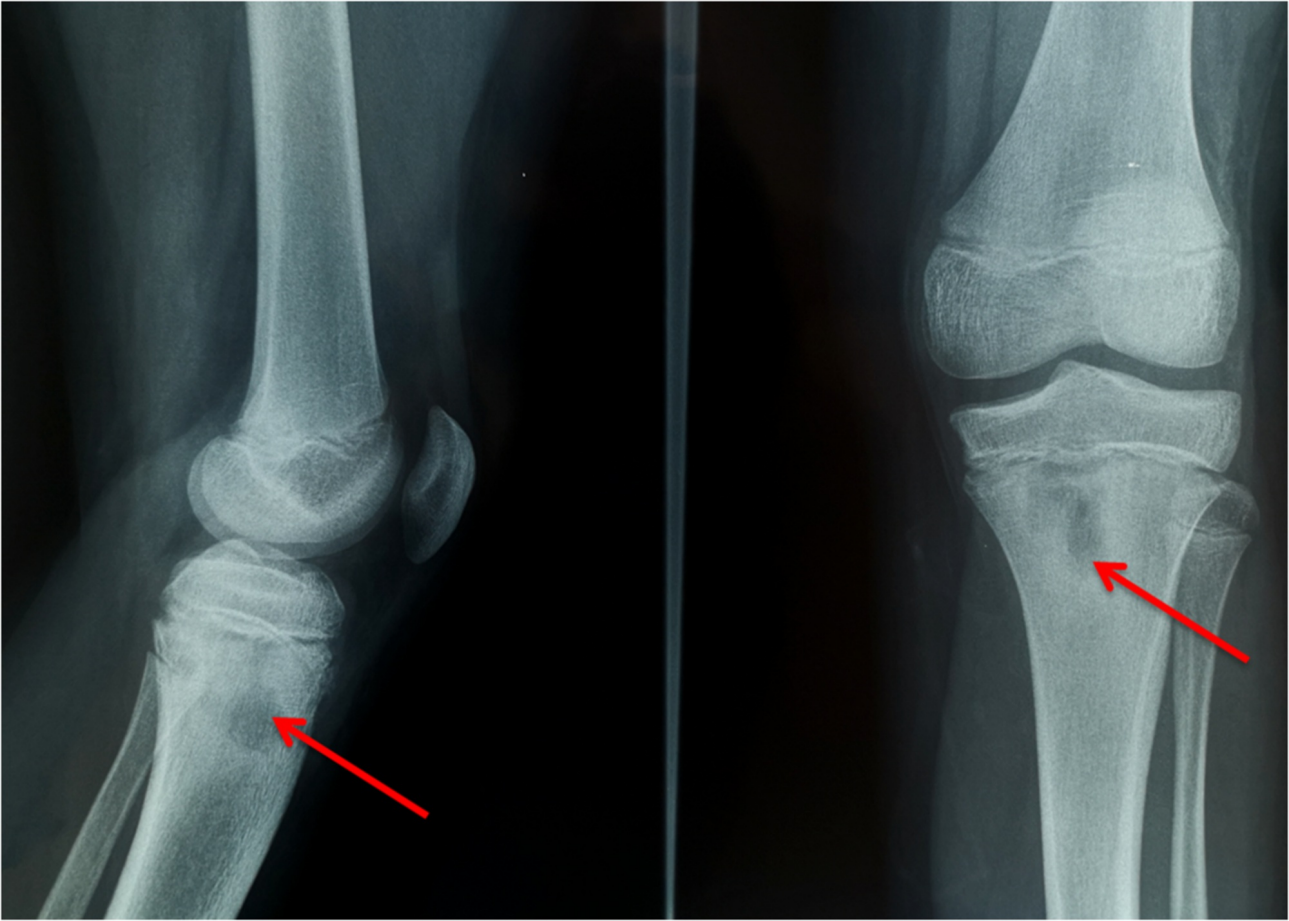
Knee radiographs at two years follow up: osteomyelitis of the proximal tibia (red arrows)
